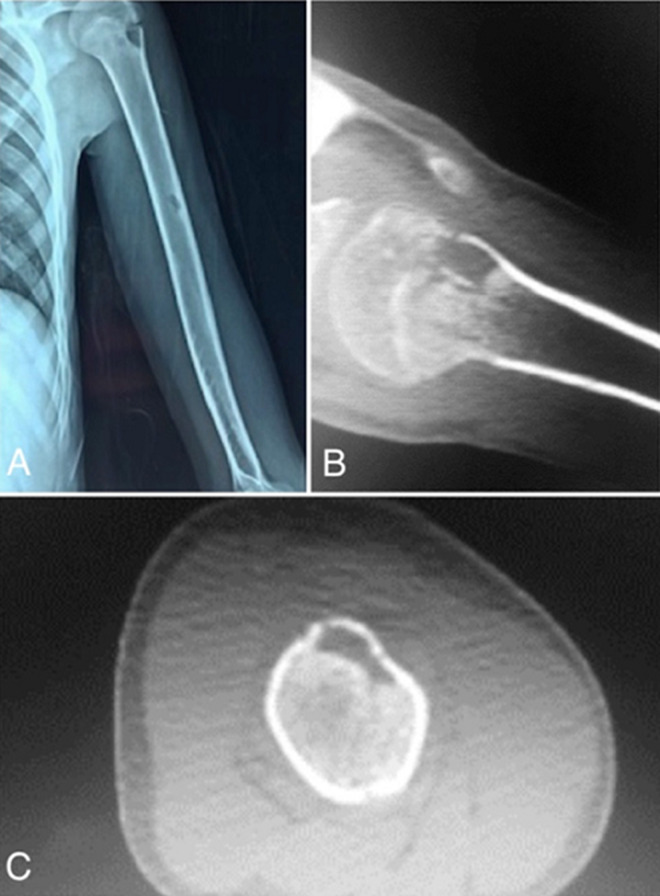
X-ray (A) and CT scan (B, C) of the left humerus showing small lytic diaphyseal and metaphyseal lesions, surrounded by marginal sclerosis
